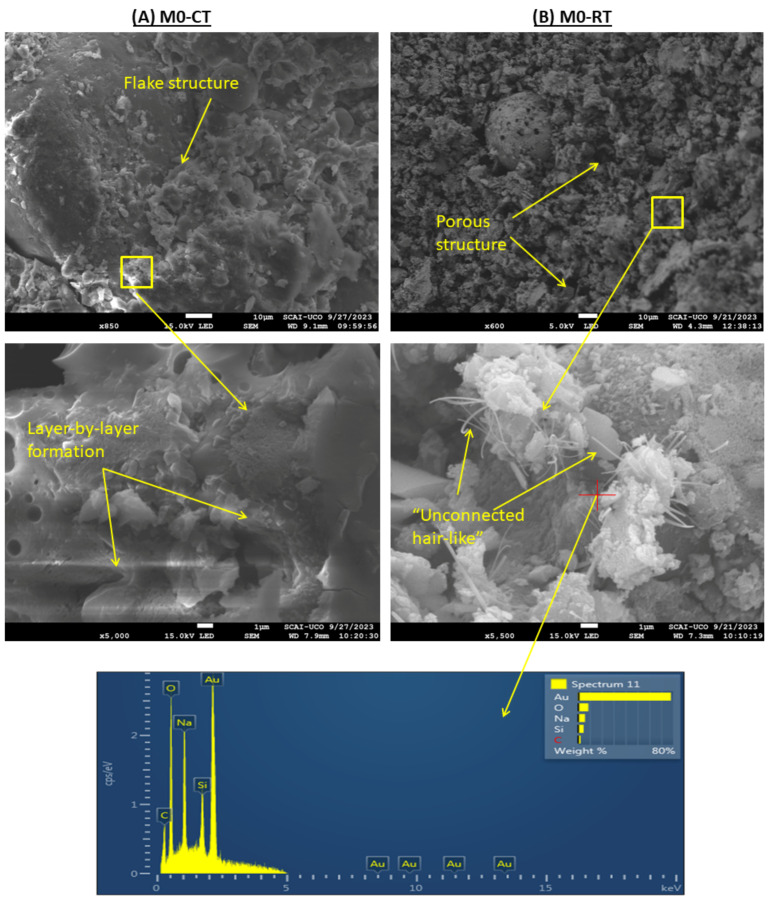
SEM and EDS of M0 under CT (A) and M0 under RT (B): the presence of Au (from the coating applied for SEM imaging) and C (originating from the alkali-activated material derived from coal fly ash) corresponds to the influence of the research method, not to the intrinsic composition of the sample.
